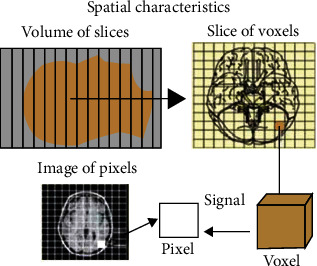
Spatial characteristics of MRI.
